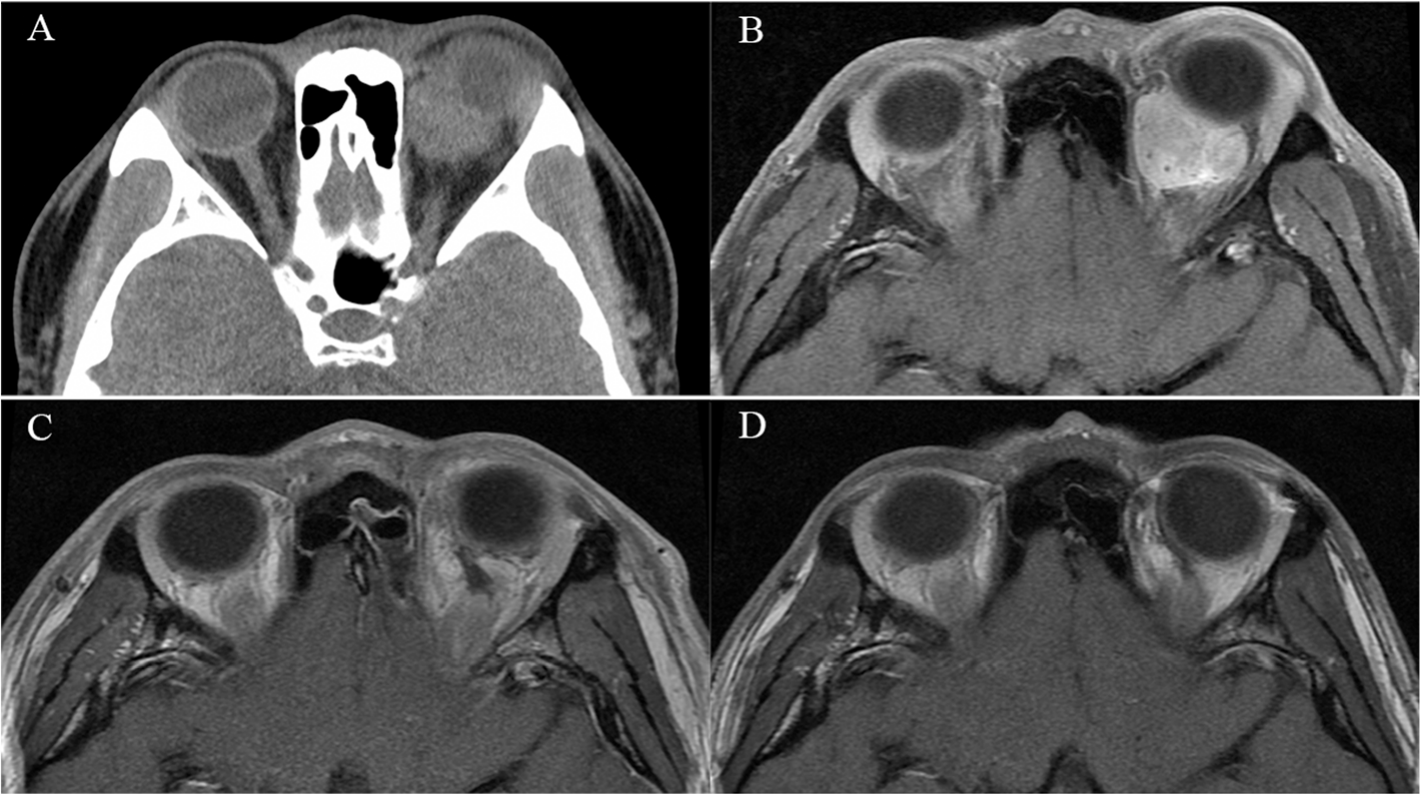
Case 1. The patient was a 23-year-old with a chronic disease process and left exophthalmos for more than 10 months. a, b: CT and MRI showed that the tumor was located in the muscle cone behind the eyeball and compressed the optic nerve. c, d: After the Transfronto-orbital approach was given to the craniotomy, the MRI re-examinations immediately after the operation and 1 year after the operation showed that the tumor was removed completely without recurrence, and the optic nerve was protected. There was no recurrence after 5 years of MRI follow-up
